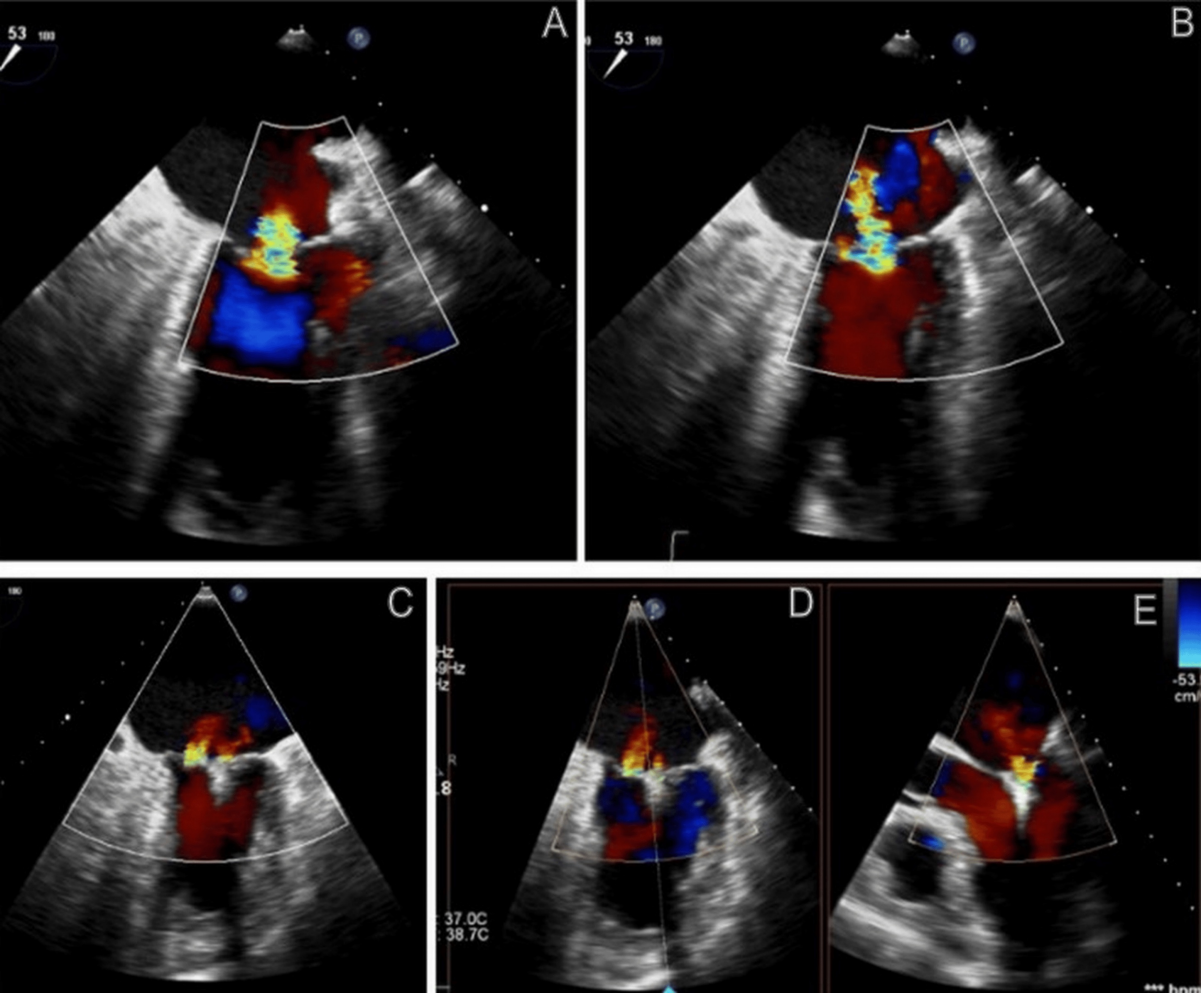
Color Doppler TEE (A, B) Color Doppler TEE showing severe mitral regurgitation before clip deployment. (C, D, E) Color Doppler TEE images demonstrating the reduction of mitral regurgitation after clip placement TEE: transesophageal echocardiography
